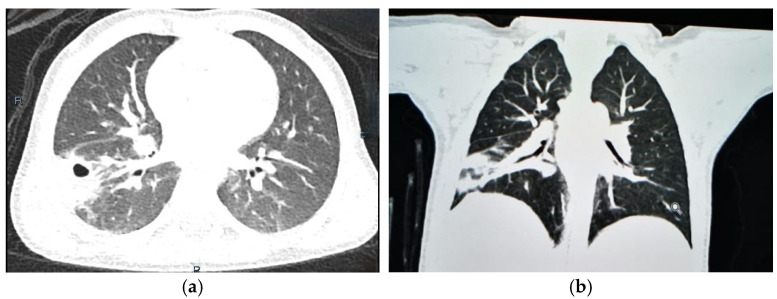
CT scan of 2-year-old male child with pulmonary abscess, demonstrating marked reduction in hydro-aeric cavity in right lower lobe, measuring 22 mm × 22 mm, without associated cystic lesions; (a) transverse plane; (b) coronal plane.
